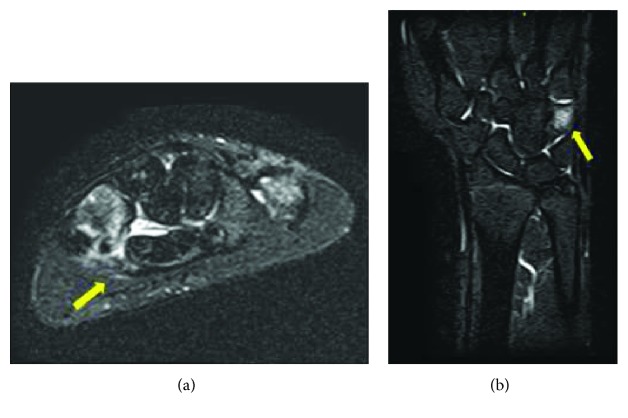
Magnetic resonance imaging (MRI) images. (a) Axial T2-weighted STIR image. (b) Coronal T2-weighted STIR image. Intensity changes around the hamate hook suggest inflammation (indicated by arrows).
